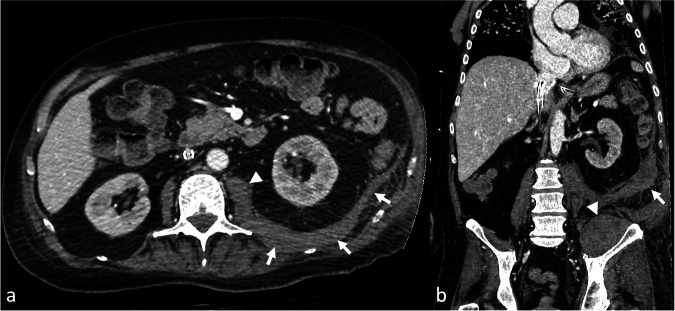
Axial (a) and coronal (b) computed tomography (CT) images in the venous phase of a 66-year-old male veno-venous (VV) extracorporeal membrane oxygenation (ECMO) supported patient show an extensive left retroperitoneal hematoma (arrows) extending to the ipsilateral psoas muscle (arrowhead). A subsequent angiography did not identify any active bleeding source, as the hemorrhage had spontaneously resolved
